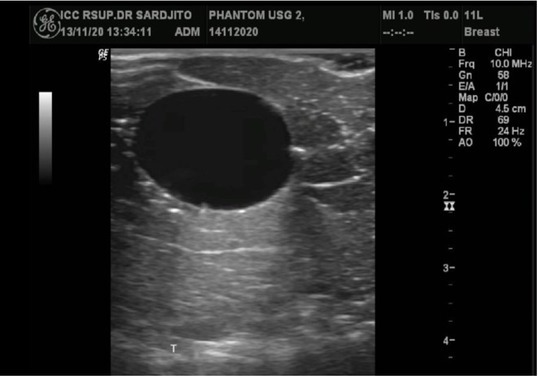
A water-filled balloon was used to mimic a cystic lesion. This ultrasound image demonstrated the cystic lesion as a well-defined, oval-shaped anechoic area with posterior enhancement.
